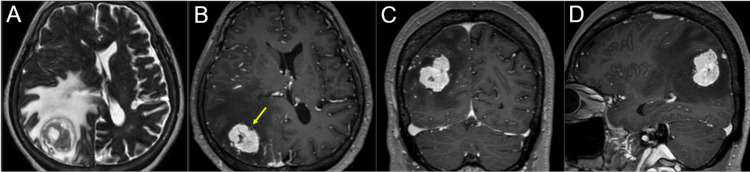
Magnetic resonance images before 7-fraction stereotactic radiosurgery. The images show T2-weighted image (WI) (A); contrast-enhanced (CE) T1-WI (B-D); axial views (A, B); coronal view (C); and sagittal view (D). (A-D) All images were co-registered on dedicated software and are shown in the same magnification and coordinates. A solid and irregular-shaped mass lesion (arrow in B) in the right parietal lobe is associated with massive peritumoral edema and mass effect.
